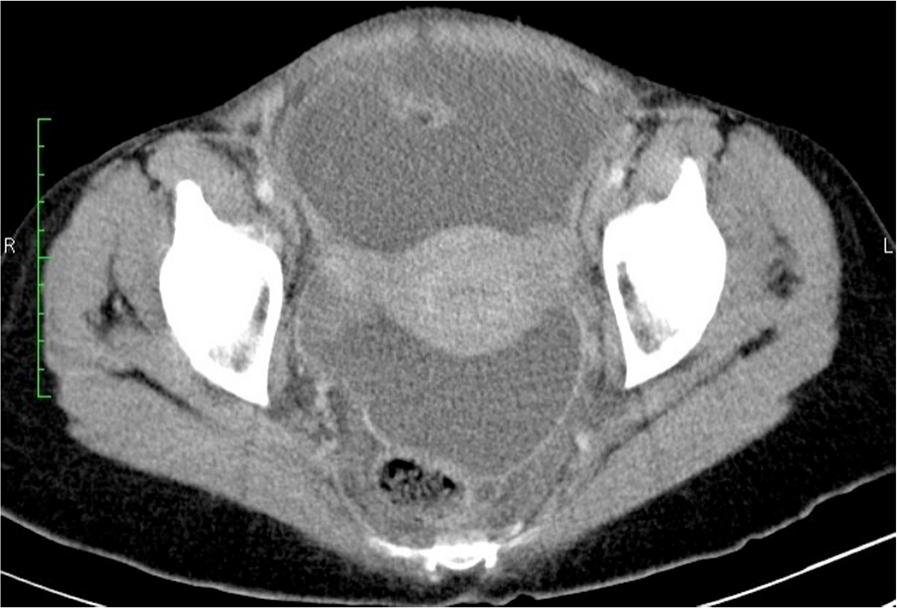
Contrast Enhanced CT of the pelvis. Moderate ascites with smooth thickening and strong enhancement of the peritoneum.
